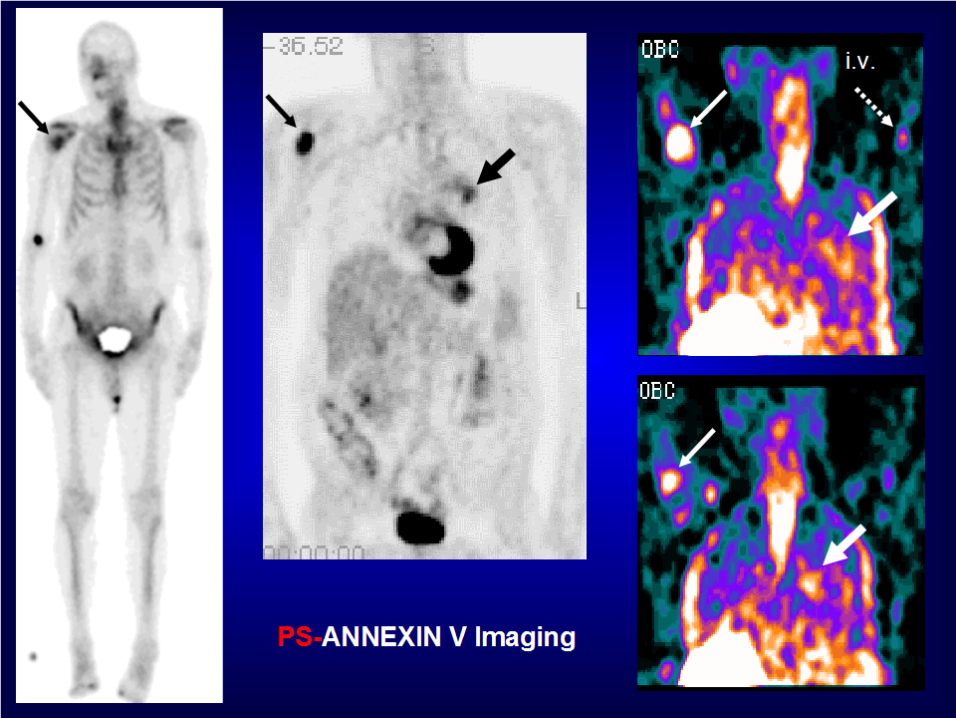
PS-annexin V imaging (North American Scientific 2020 - Phase II-III - Clinical Trial - Antwerp 2003). A patient with a left para-hilar NSCLC (large black arrow) who presented with a bone metastasis in the right upper humerus (fine black arrow) on bone scan and ^18^FDG PET. ^99m^Tc-HYNIC-annexin V imaging showed tracer uptake in the primary tumour (large white arrow) and the bone metastasis (fine white arrow) as soon as 24 h after the first course of chemotherapy. i.v., intravenous injection (dashed arrow).
